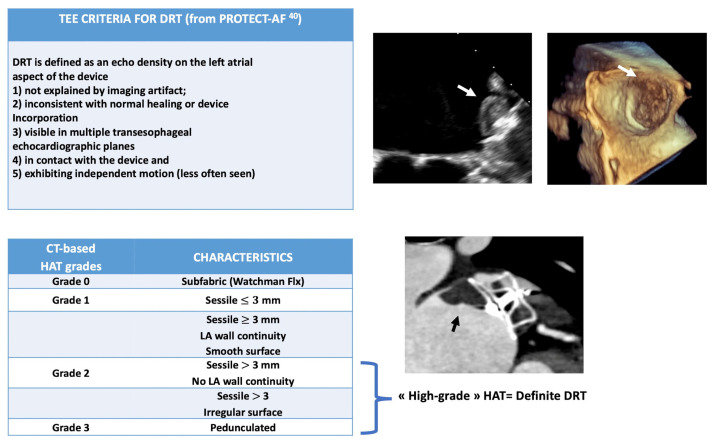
DRT Criteria for TEE and CT imaging modalities. TEE = Transesophageal Echocardiography; CT = Computed Tomography; HAT = Hypoattenuated Thickening; LA = Left Atrial; DRT = Device-Related Thrombus.
